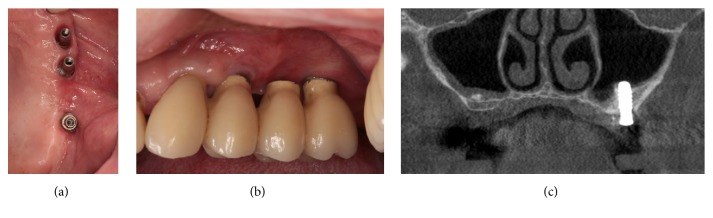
Two months after the closure of OAF. (a)-(b) Clinical views. The oroantral fistula remained successfully closed. (c) Cone-beam computed tomography image. The left sinus was totally healed.
